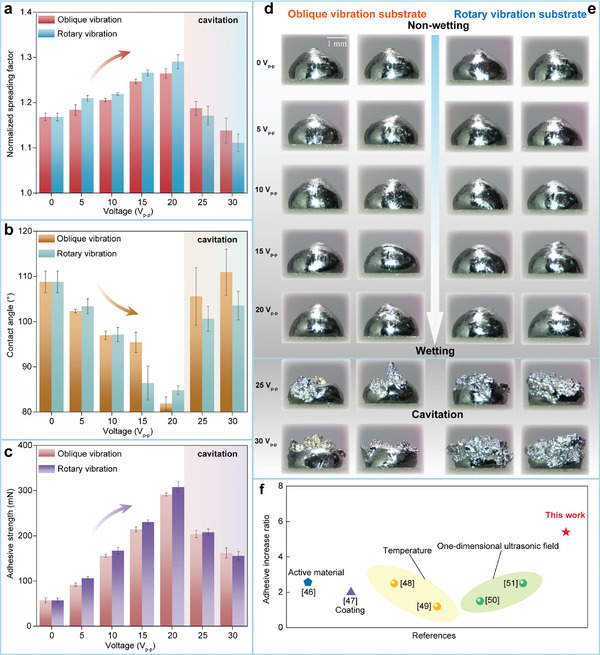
Wettability and adhesive strength of the solidified metal droplets. a) Normalized spreading factor of the solidified metal droplets versus the exciting voltage. b) Contact angle of the solidified metal droplets versus the exciting voltage. c) Adhesive strength versus the exciting voltage. d, e) The optical images of the solidified metal droplets under the treatment of the 2D ultrasonic field. f) Comparison of this work with other strategies for enhancing adhesion strength.
